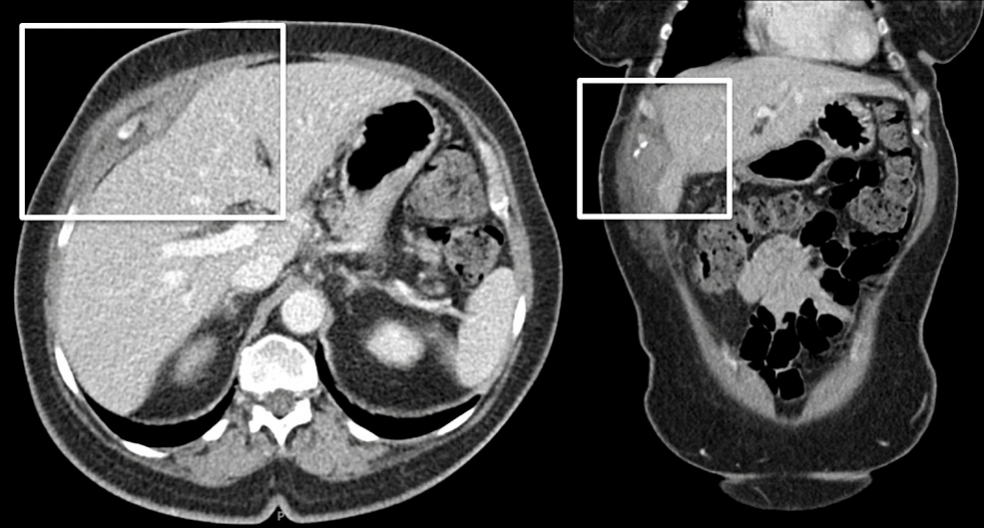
One-month follow-up CT showing interval improvement of the collection and removal of the catheter
